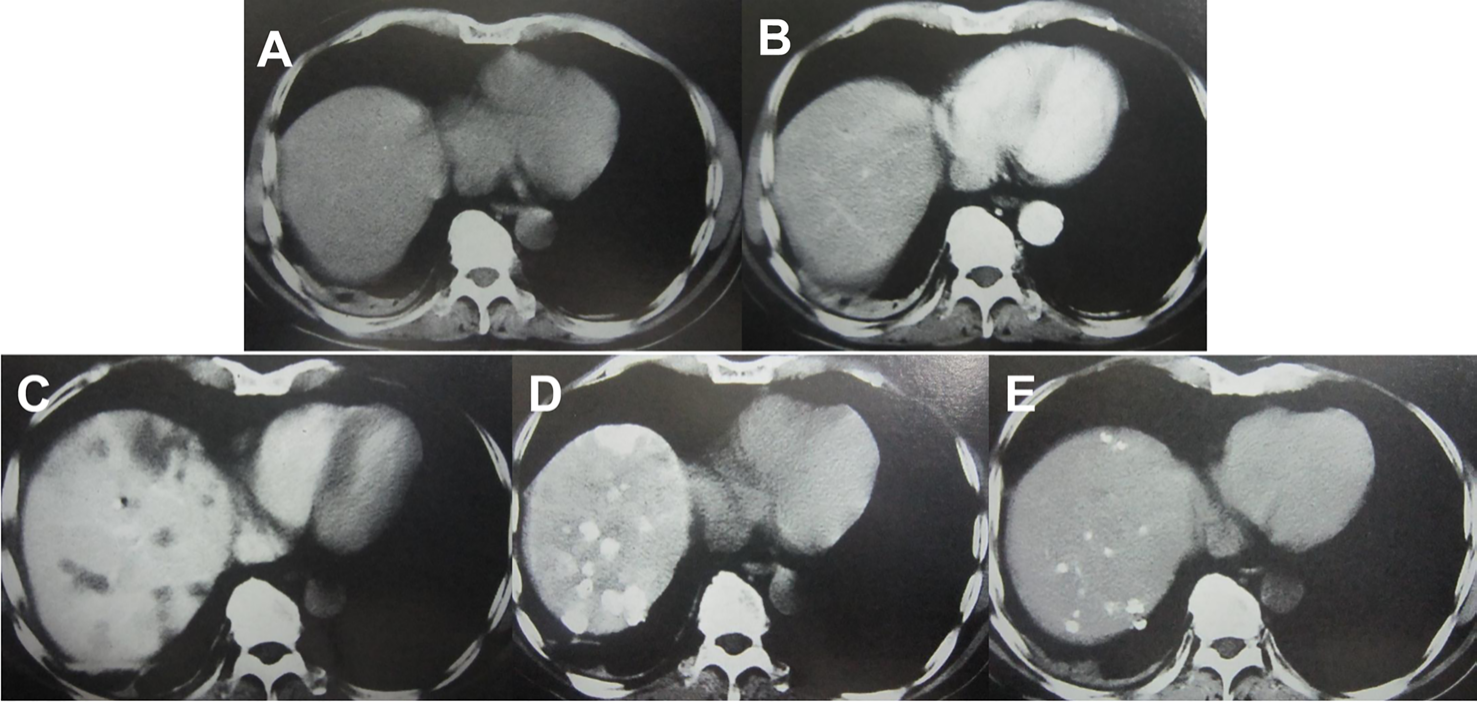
Imaging examination of an HCC patient with a progressively elevated AFP level. (A) CT plain scan showed no lesions in the liver; (B) A contrast-enhanced CT scan failed to reveal any lesions in the liver; (C) CTAP showed intrahepatic low-density lesions; (D) CTHA showed intrahepatic high-density lesions; (E) Lp-CT showed multiple intrahepatic lipiodol deposits after 3 weeks.
